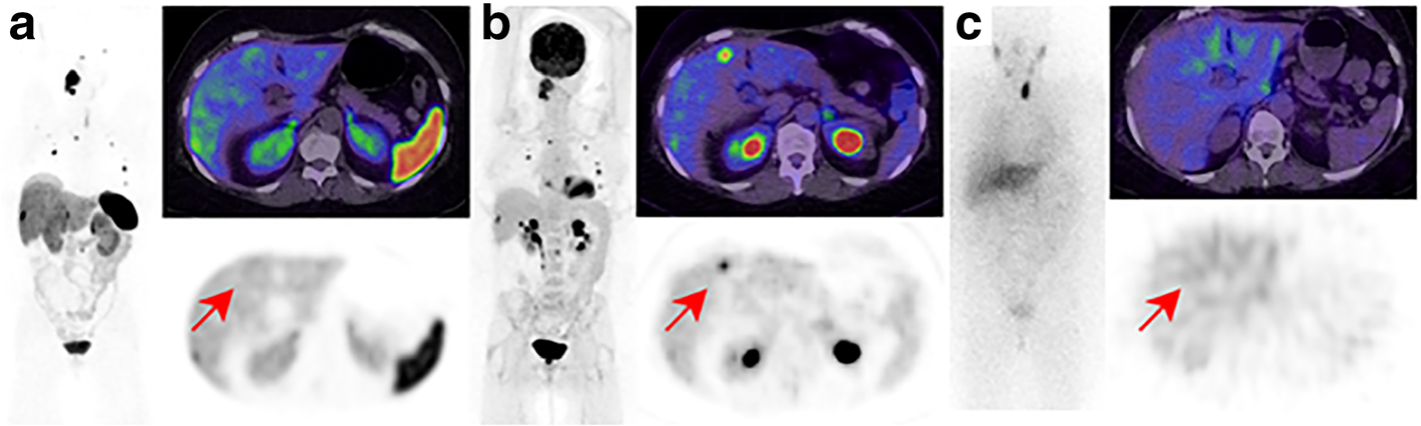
65 year-old female with negative germline mutation testing. The patient had a right glomus vagale with metastatic disease to lymph nodes, bone and liver evident on Ga-68 DOTATATE (a), F-18 FDG (b) but not I-123 MIBG (c). One of the hepatic metastases, however, was seen on FDG but not on other imaging modalities (*arrow*). The lower physiologic background activity on FDG may result in increased detection rate compared to DOTATATE in some patients
